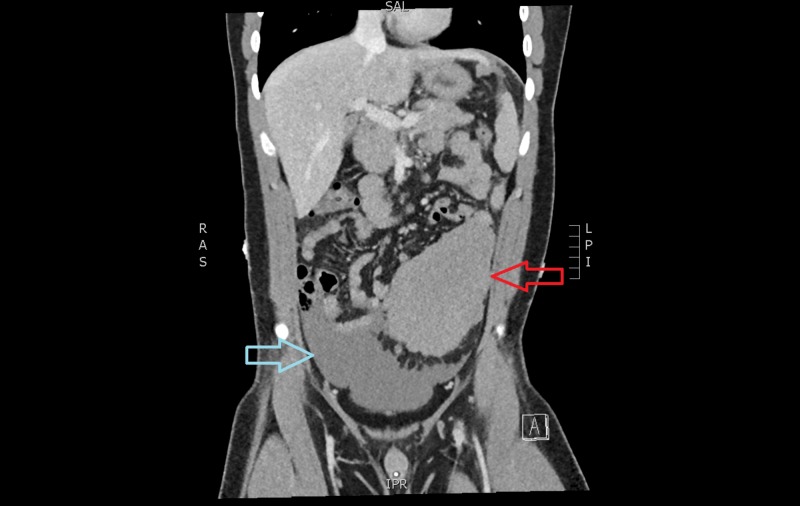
Computed tomographic angiogram (in venous phase) showing caudal migration of the heterogeneous mass and increased haemoperitoneum Red arrow: caudal migration of heterogeneous mass Blue arrow: increased haemoperitoneum
